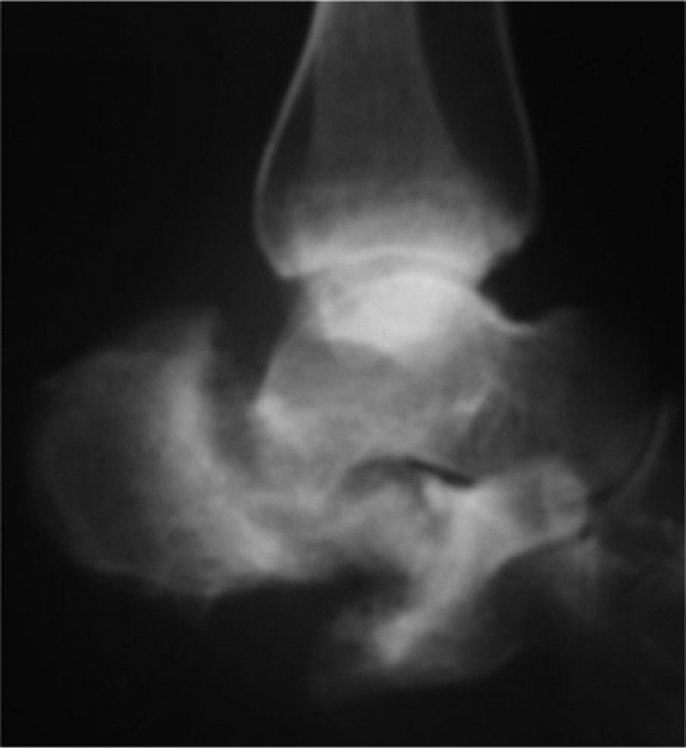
Plain radiographic lateral view showing bone resorption and collapse of the anterior and medial tuberosity of the calcaneus as well as proximal displacement of the greater tuberosity.
